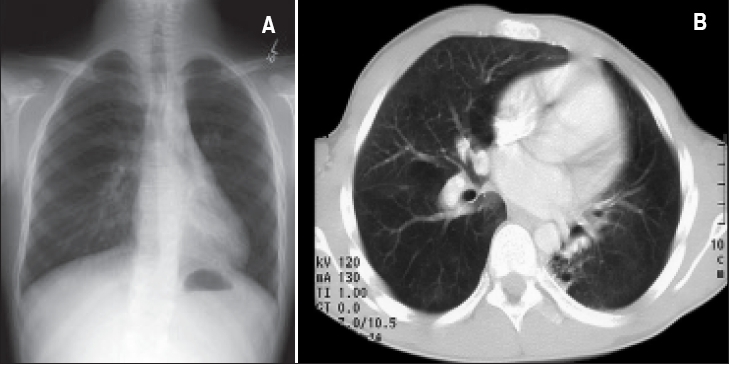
A patient with TB infection proved by lung biopsy and sputum culture and LLL bronchiectasis. A. Chest C-ray: Left lower lobe showing evidence of airway disease with bronchiectasis, bronchial wall thickening and minor peribronchial infiltrate. B. CT chest: Bronchiectatic changes in the left lower lobe, superior and anterior segments. Some areas of fibrosis with contraction of the left lung.
